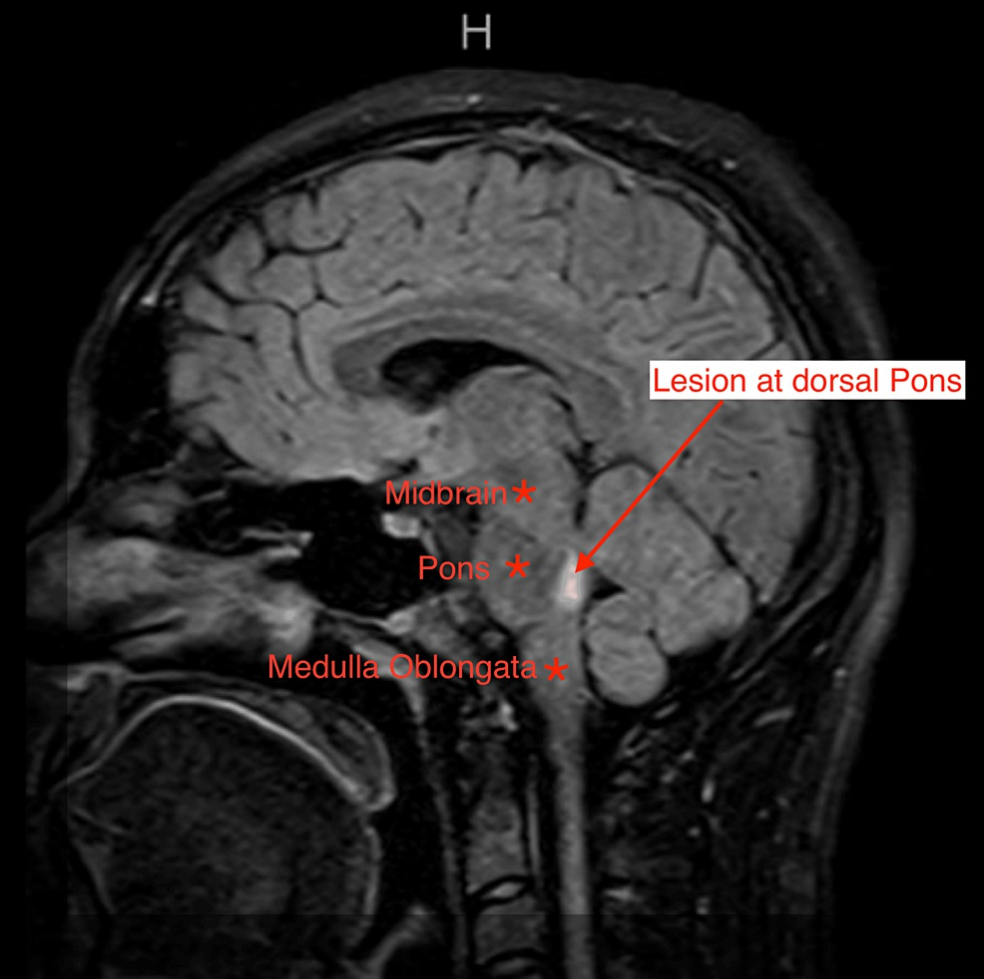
MRI brain FLAIR-weighted image lesion in sagittal view Sagittal view MRI brain FLAIR-weighted image depicting a hyperintense lesion located at the level of the dorsal pons (indicated by the red arrow). The lesion appears as a bright area against the surrounding tissue, suggestive of increased fluid content or inflammation MRI: magnetic resonance imaging; FLAIR: fluid-attenuated inversion recovery
